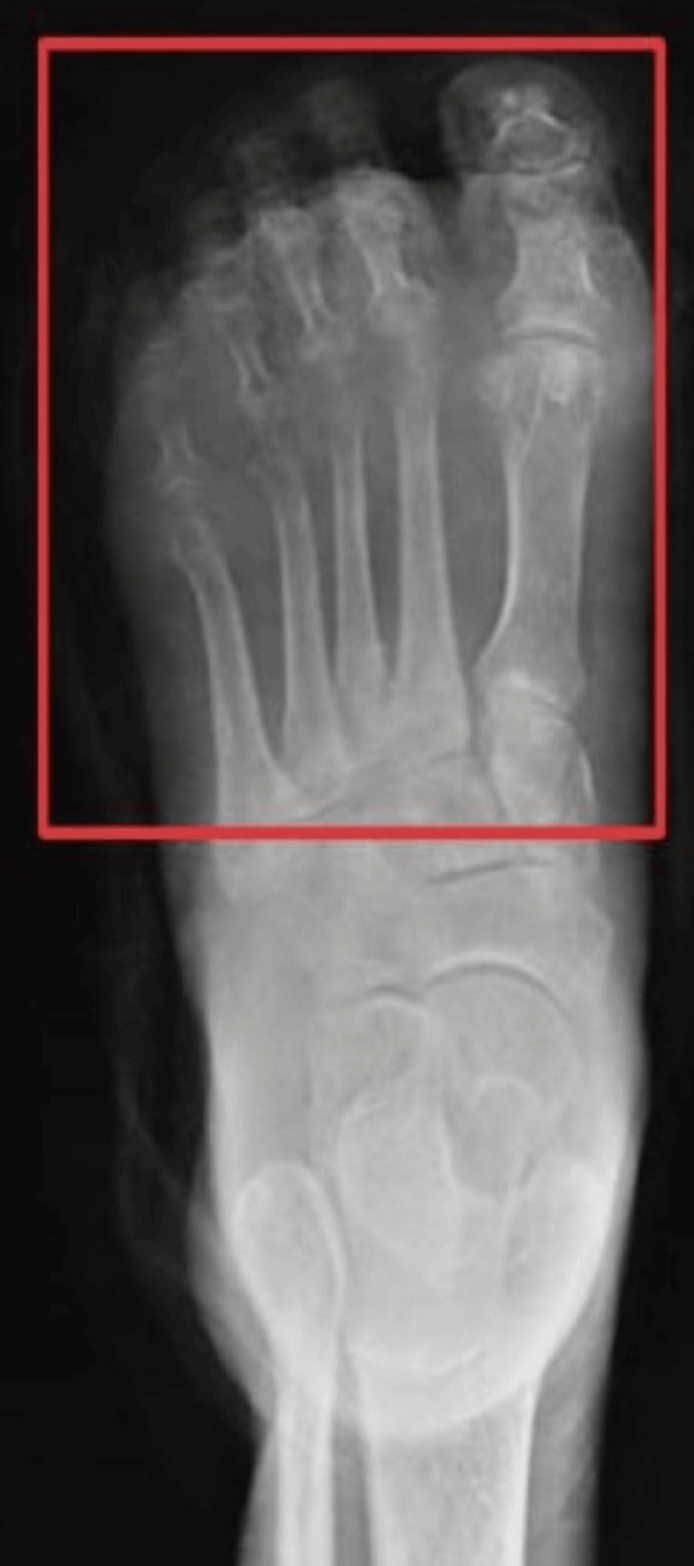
Radiograph of the left foot showing osteophytes
